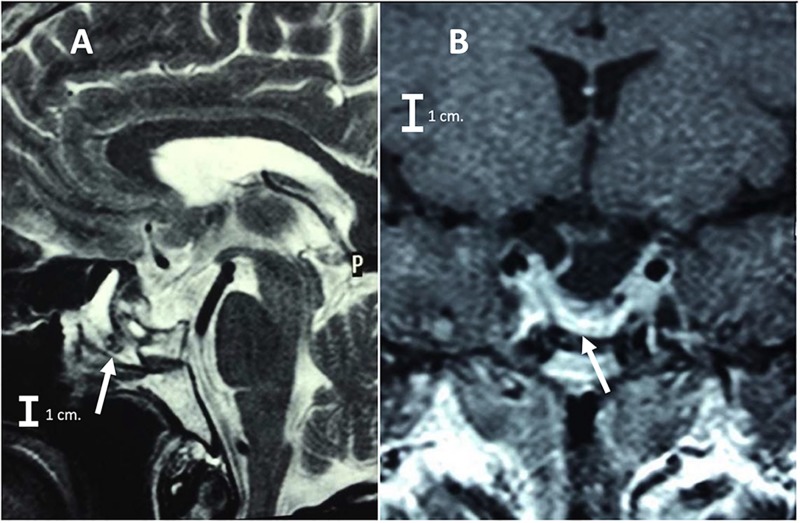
(A) Postoperative coronal brain MRI T1 signal respectively, showing the gross total resection of the Macroadenoma and the reconstruction of the sellar floor (arrow) with the bilaminar chitosan scaffold, (B) sagittal brain MRI T2 signal (arrow showing also the reconstruction of the sellar floor with the bilaminar chitosan scaffold).
